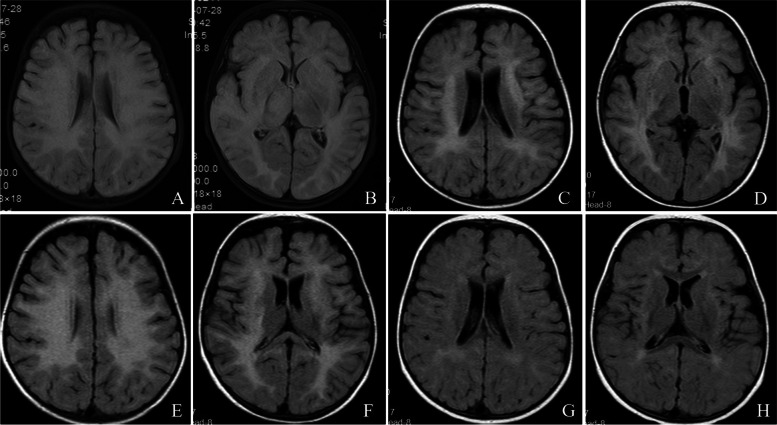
Brain MRI evolution of patient 1 at onset and during follow-up. Images of extensive and confluent bilateral white matter involvement and basic symmetry of lesions for the first episode (A, B). Bilateral white matter lesions were reduced and new lesions were found in the right occipital cortex (C, D). Bilateral cerebral hemispheres and centrum semiovale lesions increased (E, F). Although image lesions were reduced and stabilized after immunotherapy (G, H), the child showed cognitive impairment and epilepsy
